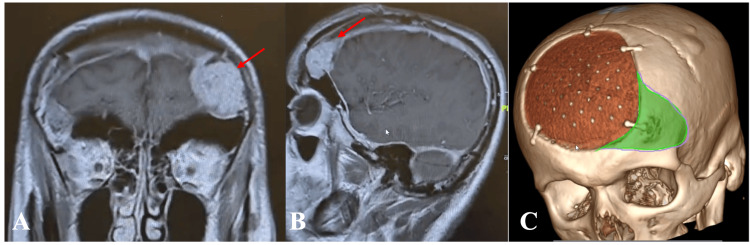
(A) Coronal and (B) sagittal preoperative images of MRI brain (T1-weighted with contrast) showing left frontal tumor (red arrow). (C) Preoperative planning images for the generation of template and PEEK implant MRI: magnetic resonance imaging; PEEK: polyetheretherketone
